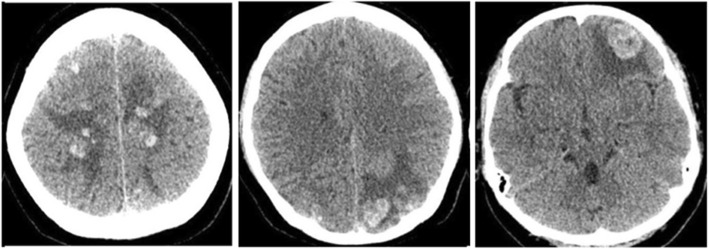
CT brain image shows multiple hemorrhagic lesions surrounded by edema
